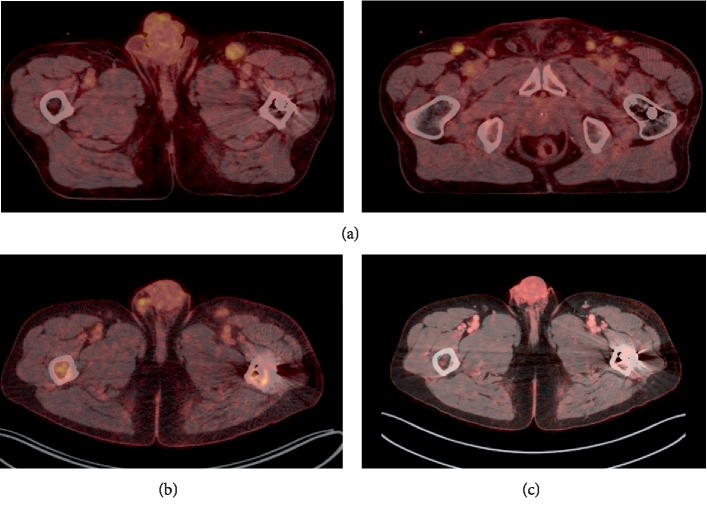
(a) PET-CT at baseline showing an enlarged lymph node in the left and right groin measuring 2.7 × 1.9 cm and 1.4 × 1.6 cm, respectively, with increased metabolic activity. Enlarged and hypermetabolic lymph nodes were also found in the mediastinum and the left side of the retroperitoneum. (b) PET-CT after course 10 showing lymph nodes in the left groin with normal size and metabolic activity. Rest of the PET-CT showed complete remission of other tumour sites. (c) PET-CT after 15 months at follow up with no signs of relapse.
